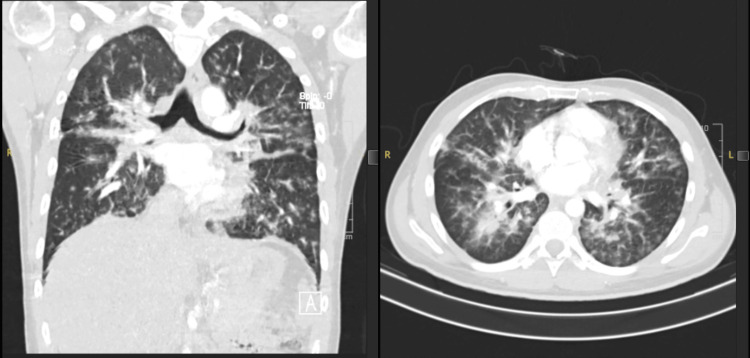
Coronal view (left) and axial view (right) of CTPA shown in the lung window CTPA done during the patient's second admission in April 2021 to exclude pulmonary embolism demonstrates multifocal, patchy, ground-glass opacities mixed with interstitial opacities seen at both lung fields, worst at the superior segment of the right lower lobe, left lingula segment, and perihilar region with atelectatic changes at both lung bases CTPA: computed tomography pulmonary angiogram
